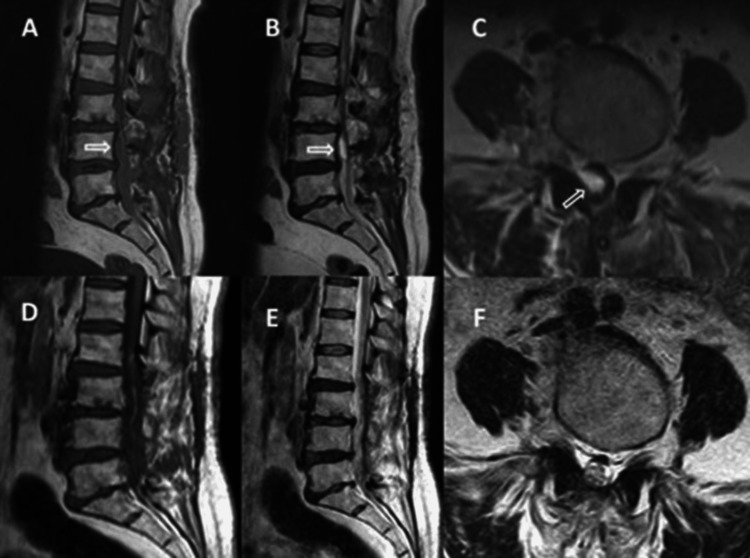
(A-C) Lumbar magnetic resonance images of the subdural hematoma. (D-F) Control lumbar magnetic resonance images one year after the surgery. (A) Isointense hematoma on sagittal T1-weighted image. (B) Hyperintense hematoma on sagittal T2-weighted image. (C) Hyperintense hematoma on axial T2-weighted image. (D) Sagittal T1-weighted image. (E) Sagittal T2-weighted image. (F) Axial T2-weighted image.
